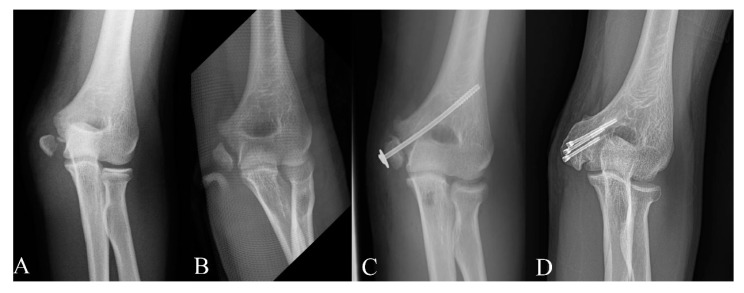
(A) Radiograph demonstrating a medial epicondyle fracture with marked displacement in a 13-year-old girl at the initial time of injury. She was treated with cast immobilization only. (B) Established medial epicondyle nonunion was seen at 6 months after injury. She had tenderness upon palpation over the medial epicondyle and pain with valgus stress. (C) Nonunion was still seen at 1 year after open reduction and internal fixation with a screw and washer. (D) Bony union was achieved at 6 months after revisional osteosynthesis with an autologous bone graft.
